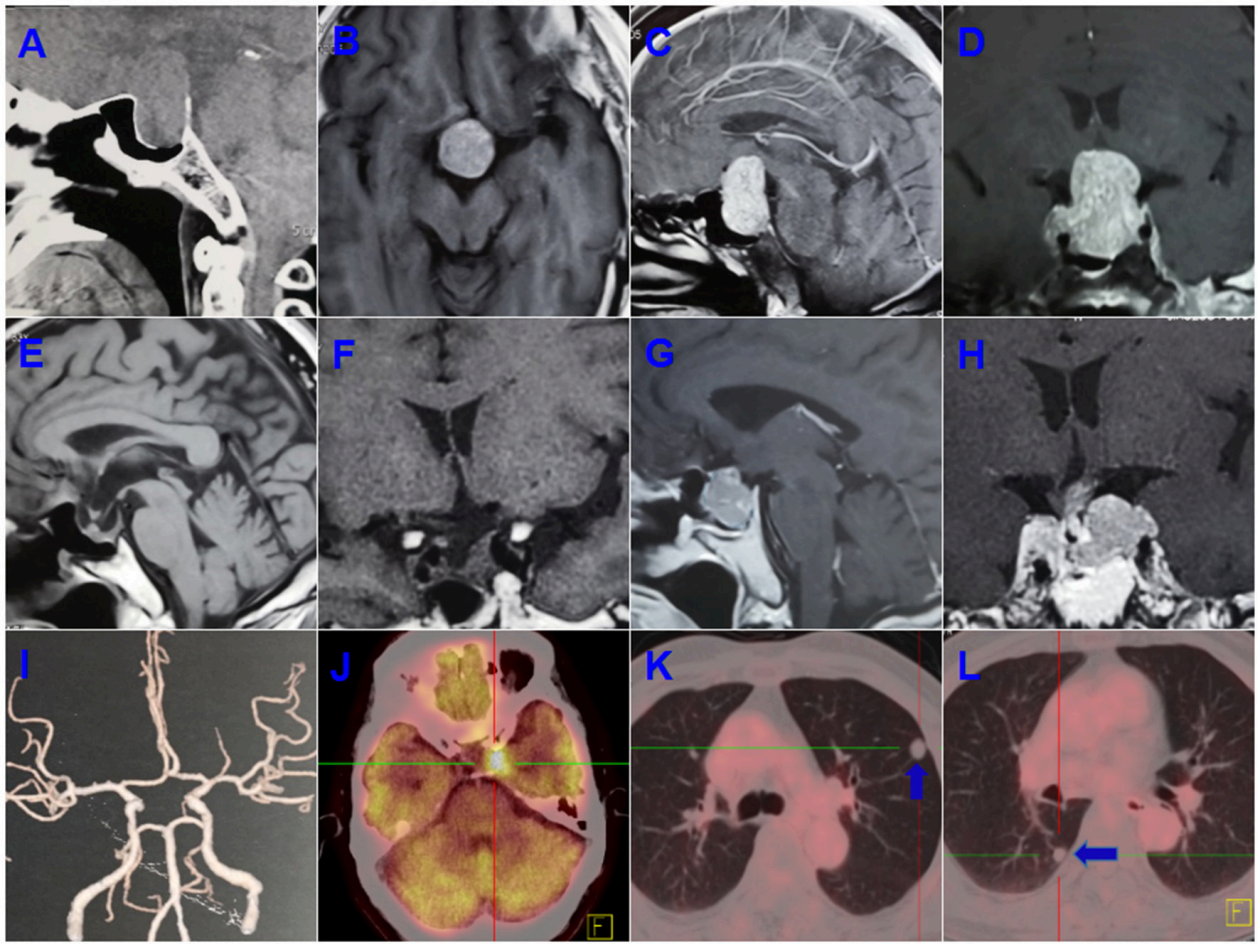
Preoperative computed tomographic (CT) scanning revealed a large mass lesion in the sellar region (A). Magnetic resonance imaging (MRI) also demonstrated a large lesion located in the sellar region, with heterogeneous enhancement and invasion to both cavernous sinuses (B–D). Subtotal resection was obtained after operation (E,F). A recurrent tumor was involved in the saddle fossa and left cavernous sinus (G,H). Computed tomographic angiography (CTA) ruled out a left posterior communicating artery aneurysm (I). The PET-CT showed a residual intracranial tumor in the left cavernous sinus after a second operation (J). Multiple metastatic lesions were found in the lung (K,L; blue arrow indicates the metastatic lesion).
